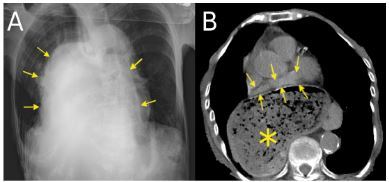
(A) Chest radiograph showing a mass shadow on the right side of the mediastinum (arrows). (B) Computed tomography of the chest in axial view showing displacement of the left atrium (arrows) by a giant esophageal hiatal hernia (stars).
